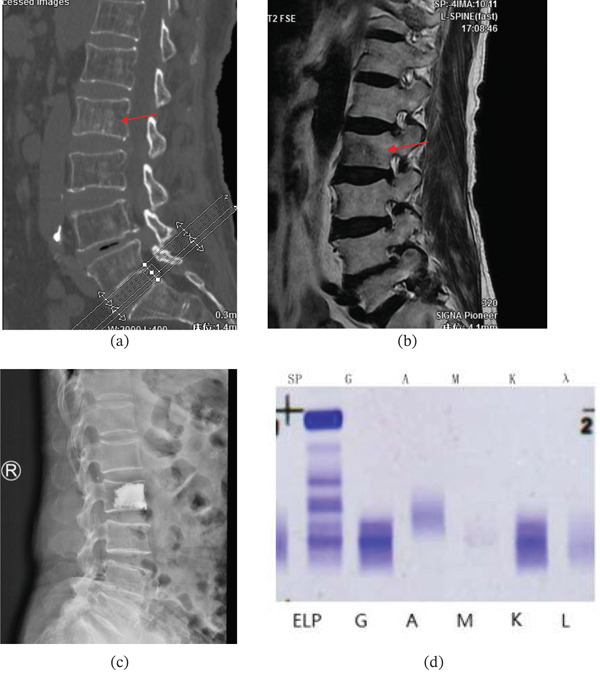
Imaging and laboratory findings of the patient in Case 4 (multiple myeloma misdiagnosed as OVF). (a) Preoperative sagittal CT showed no obvious bone destruction at L2. (b) Sagittal MRI showed signal changes in the L2 vertebral body without focal paravertebral mass or epidural extension—the absence of these malignant features paradoxically reduced suspicion for myeloma, a recognized diagnostic pitfall. (c) Postoperative lateral radiograph after L2 PVP showed satisfactory cement filling. (d) Serum immunofixation electrophoresis revealed an IgG‐*κ* monoclonal protein, confirming multiple myeloma.
